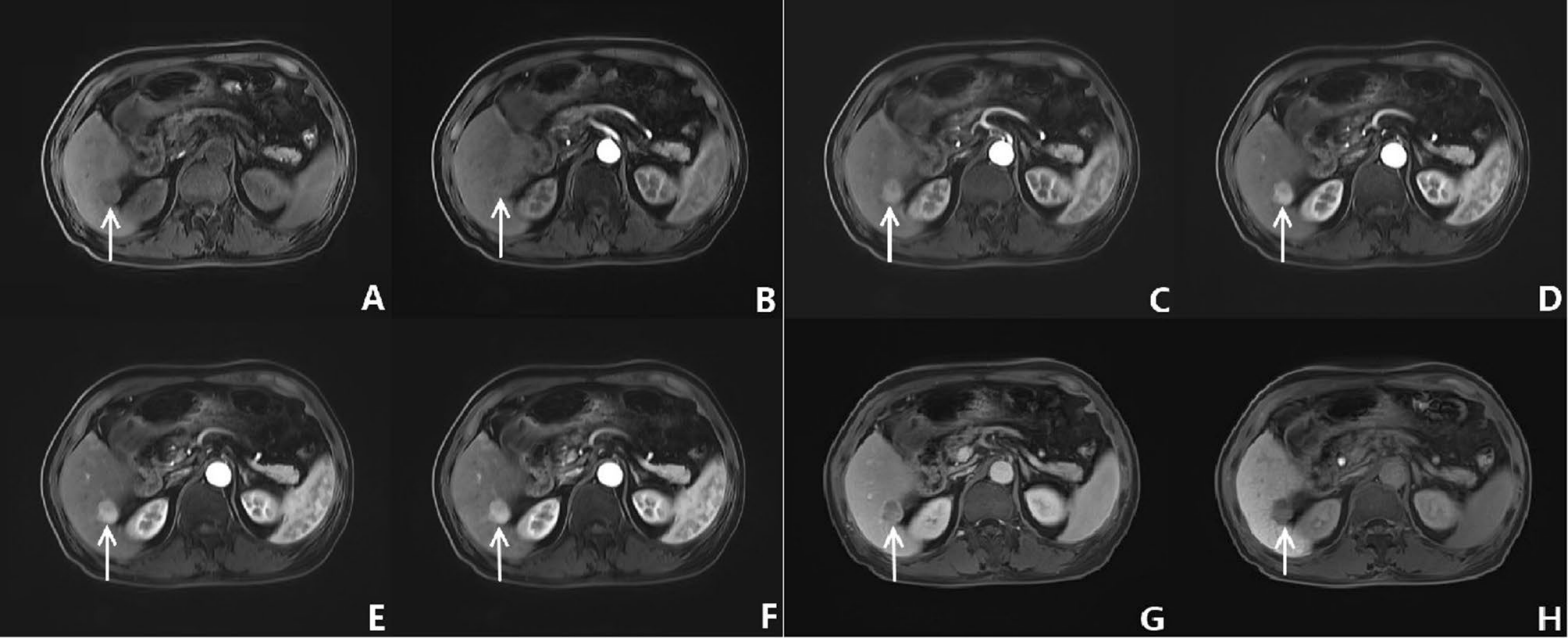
Fifty-eight-year-old man on gadoxetate acid disodium-enhanced liver MR examination using CDT-VIBE sequence. Precontrast phase (A), followed by five subphases of CDT-VIBE at (B)–(F), The portal venous phase (H) and hepatobiliary delay period (G) were shown by VIBE. MR images showed a 2.4 cm mass (white arrows) in S6/8. This mass presents peripheral arterial enhancement and washout in portal venous phase and hepatobiliary delay period. We proved this mass to be hepatocellular carcinoma by Pathologic examination. The scores of artifact degree showed that (A) was 1 point, (B) and (C) were 3 points, and (D)–(G) was 1 point. Respiratory movement artifacts were more likely to occur in the early phase of CDT-VIBE enhancement.
